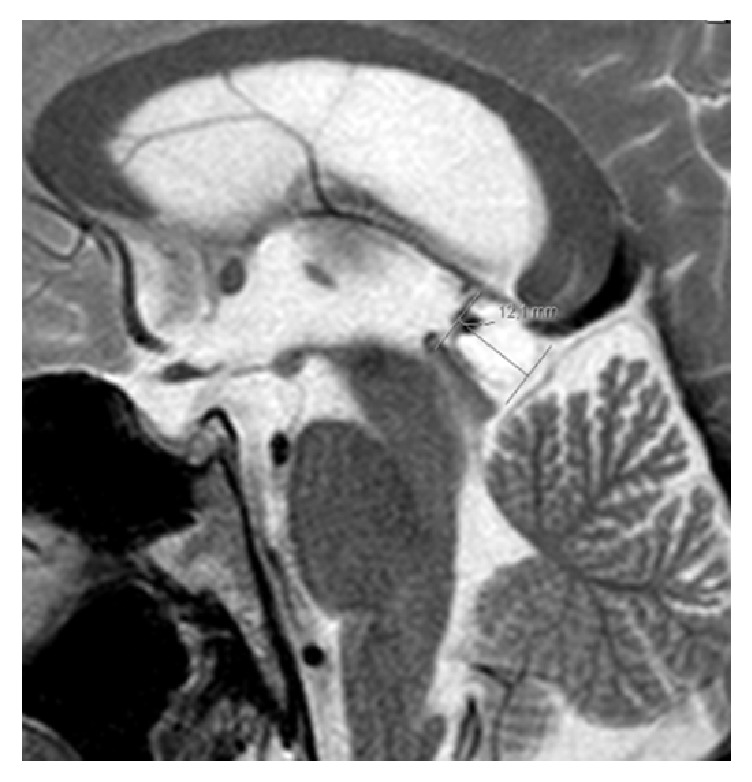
MRI of* sella turcica* at admission (August 2014): heterogeneous enlargement with peripheral contrast enhancement.
